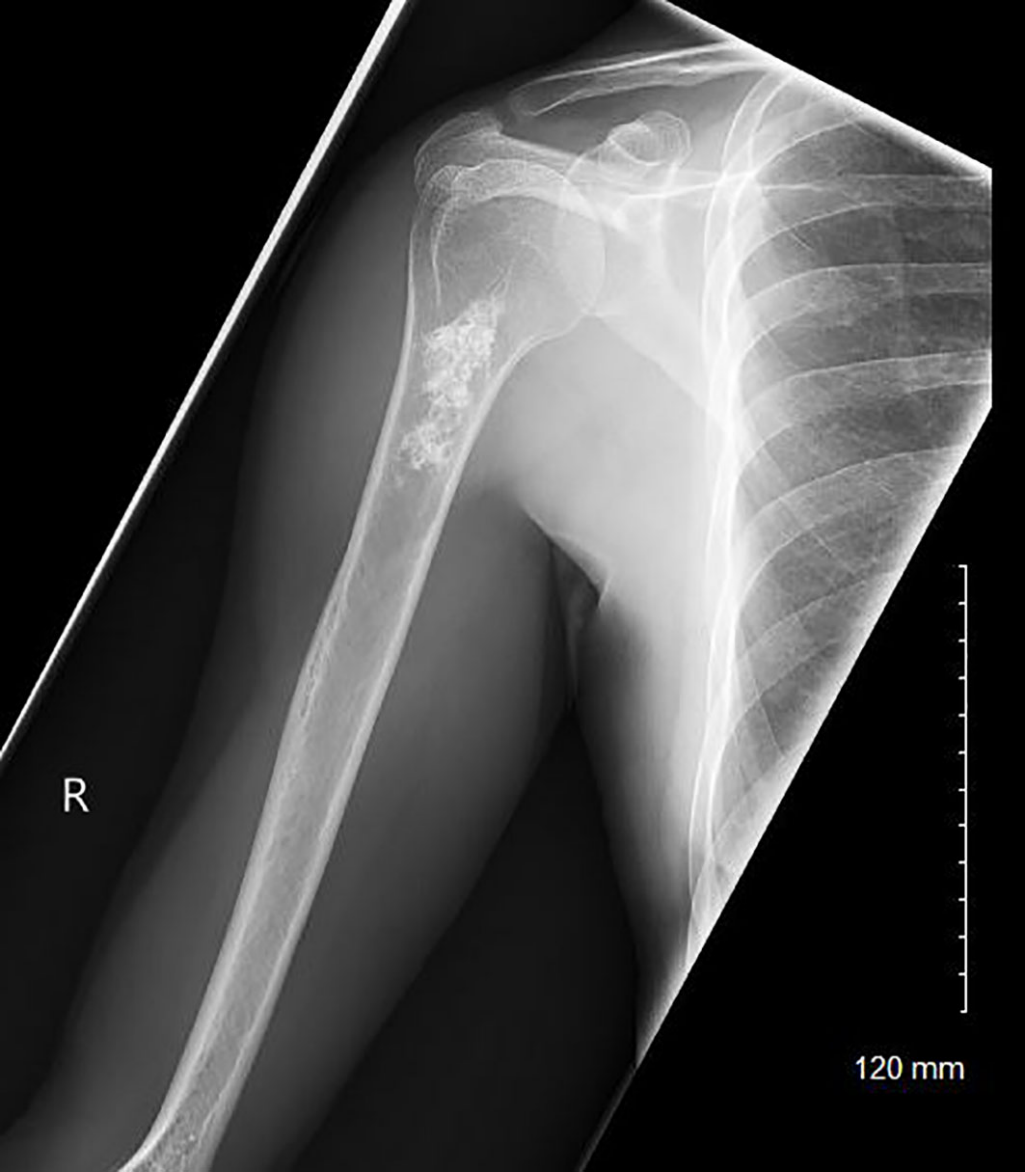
A radiograph of a patient with a low-grade chondroid lesion of the metadiaphyseal region of her right proximal humerus.
